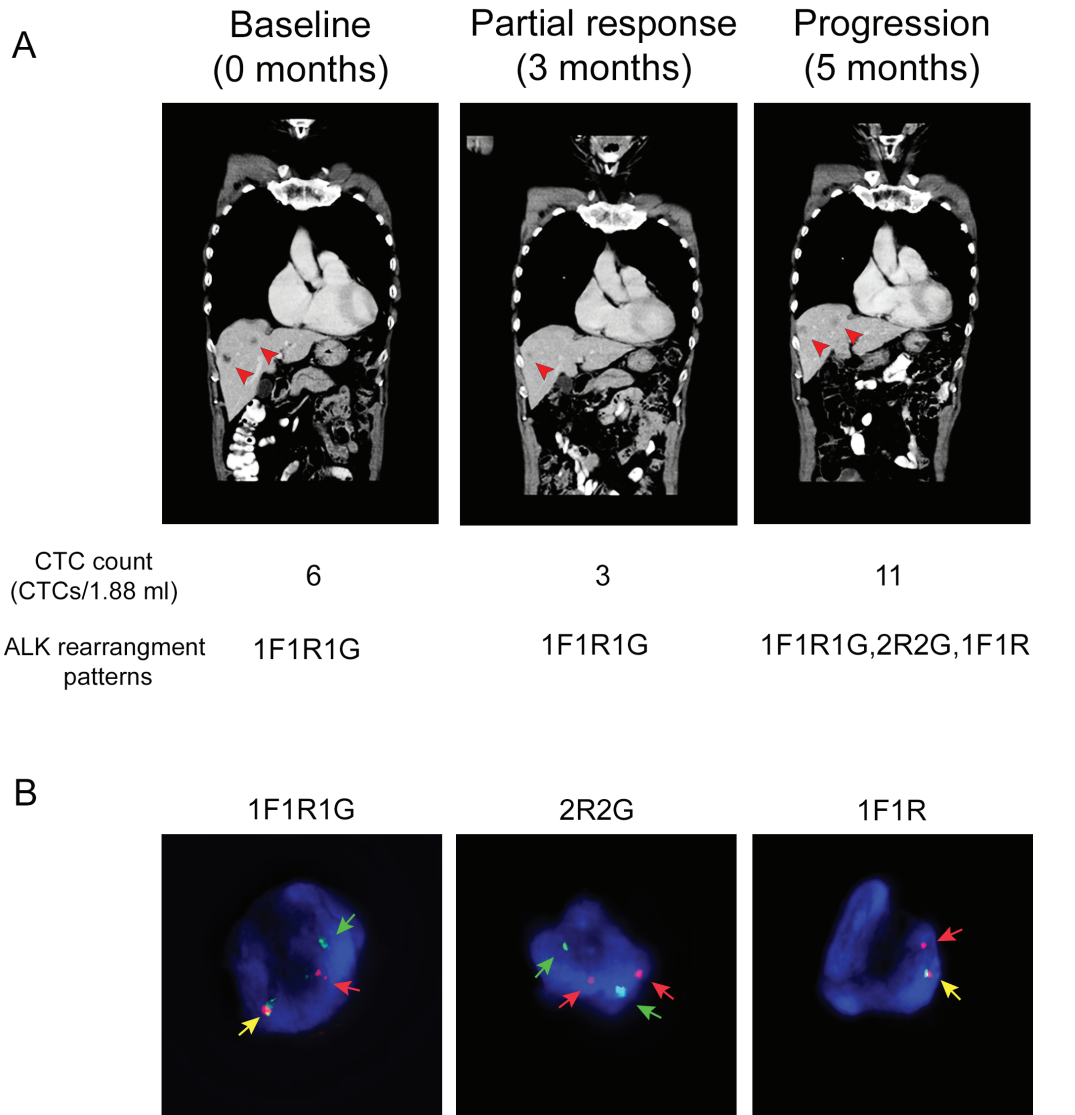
An index case suggests that ALK-rearranged CTCs could have clinical application as a diagnostic biomarker to monitor crizotinib treatment and response A. CT scan taken at baseline, partial response and progression time points showing presence of metastatic tumor in liver (arrow). CTC counts and *ALK* rearrangement patterns for each time point is indicated in the lower panel. B. Representative images showing 1F1R1G, 2R2G and 1F1R *ALK* rearrangement patterns following progression on crizotinib treatment. Yellow, red and green arrows represent fusion (F), orange (R) and green (G) fluorescent signals.
